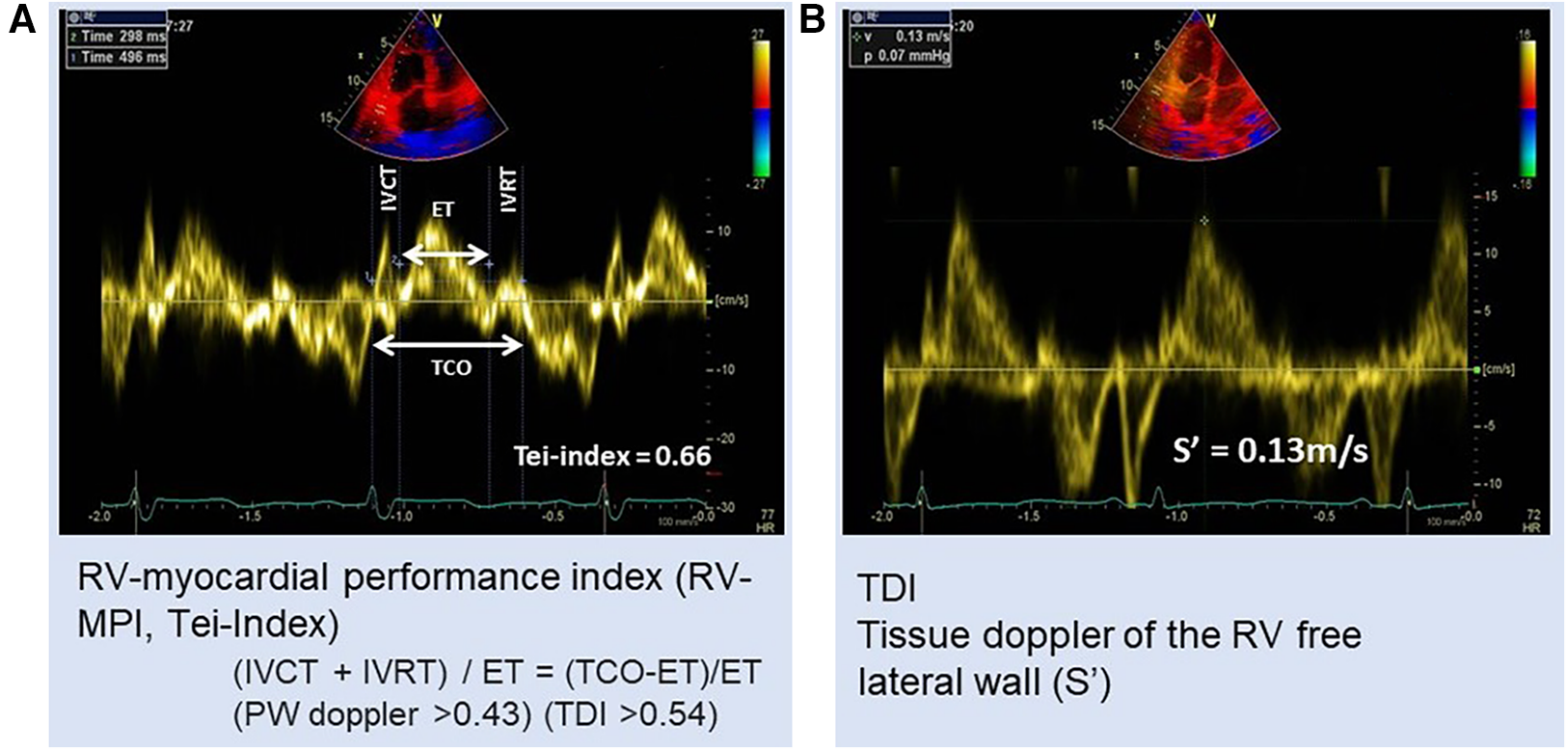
Patient with PH: (A) RV myocardial performance index (MPI) or Tei-index = 0.66 and (B) tissue Doppler velocity of RV free wall S′ = 0.13 m/s. ET, ejection time; IVCT, isovolumetric contraction time; IVRT, isovolumetric relaxation time; TCO, tricuspid valve closure to opening time.
